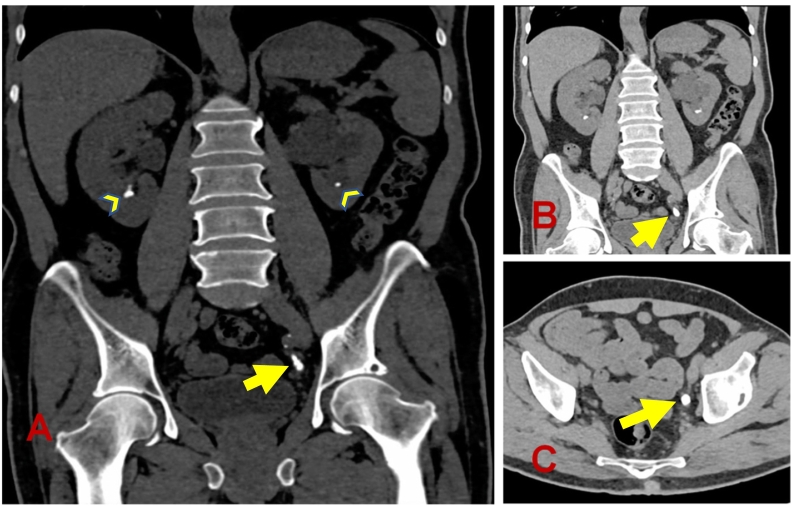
Plain CT urinary tract. Multiple left distal ureteric calculi (A-C, yellow arrows) with moderate to severe left hydroureteronephrosis. Multiple bilateral renal calculi are also noted (arrowheads). (For interpretation of the references to color in this figure legend, the reader is referred to the web version of this article.)
